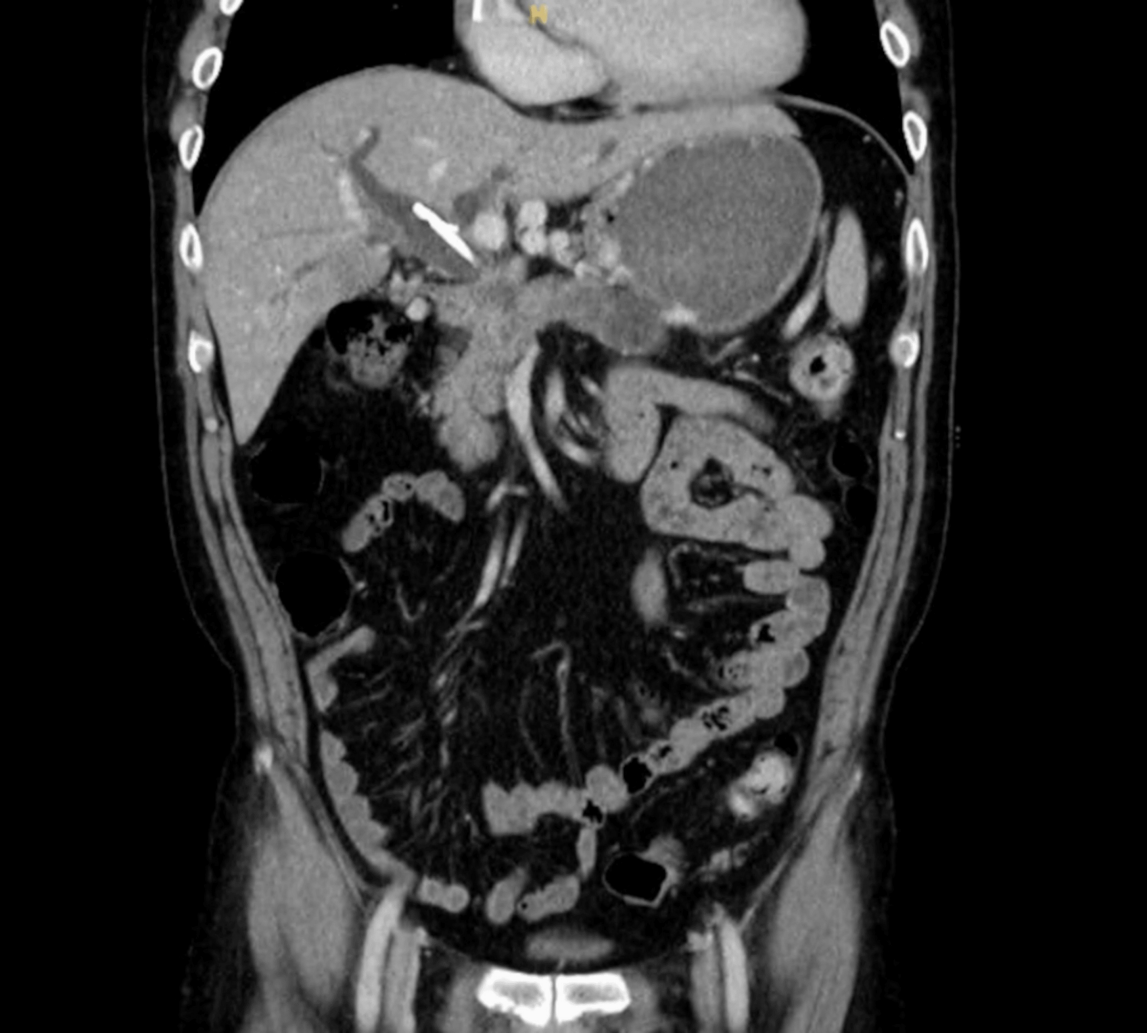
Abdominopelvic CT image of the patient’s type 1 Mutignani stricture with concurrent biliary obstruction and duodenal obstruction proximal to the ampulla CT: computed tomography
